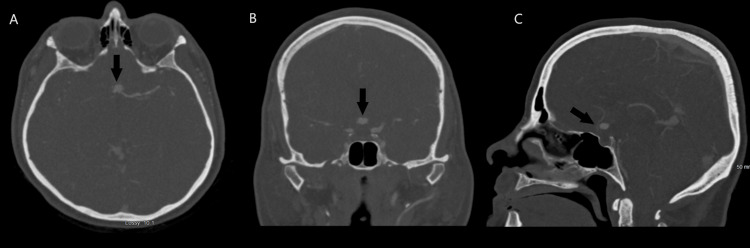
Pre-operative computed tomography angiogram of the head identifying a 7.2 x 8.1 x 5.8 mm anterior communicating artery aneurysm. Pre-operative computed tomography angiography was performed identifying the anterior communicating aneurysm. Axial (A), sagittal (B), and coronal views (C) are presented with arrows indicating the aneurysm.
